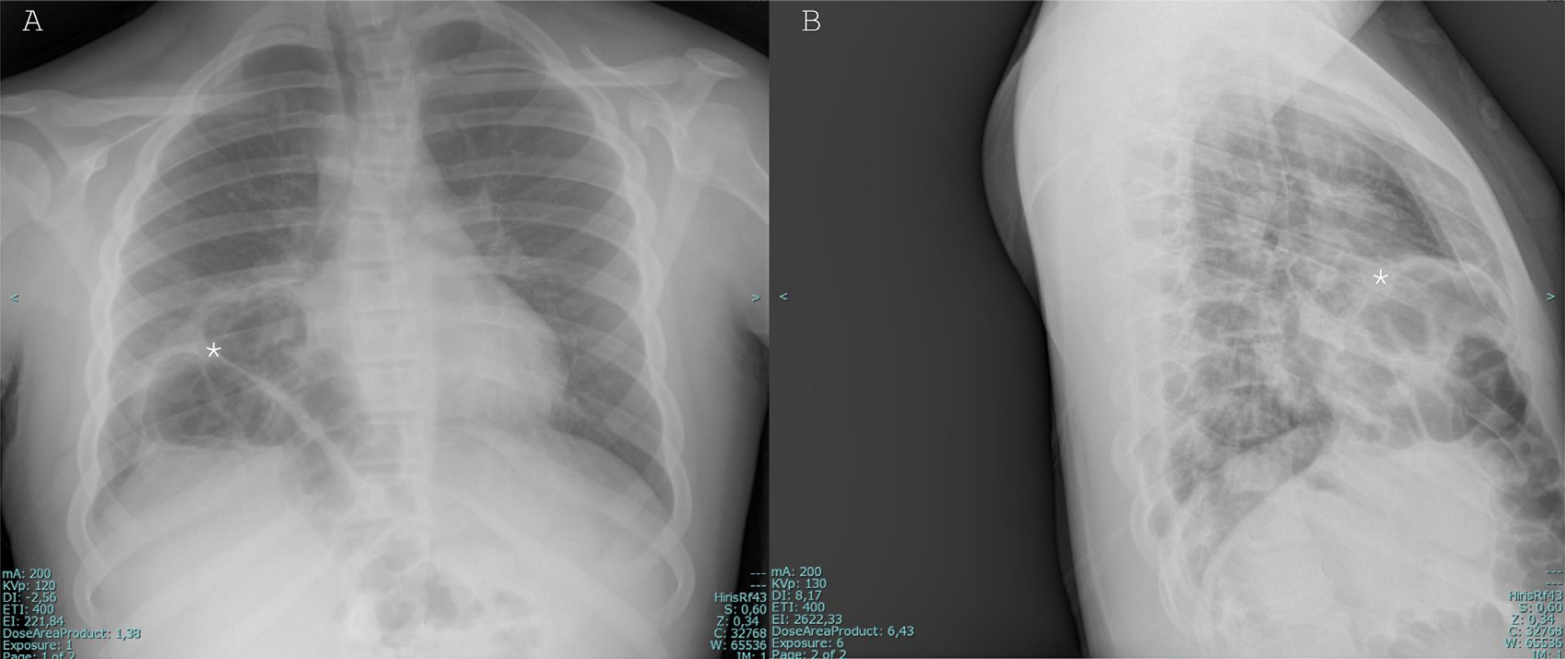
PA (A) and LL (B) projections chest x-ray showing bowel loops in the right pleural cavity. The evidence of haustras (asterisk) is consistent with herniation of trasverse colon.
